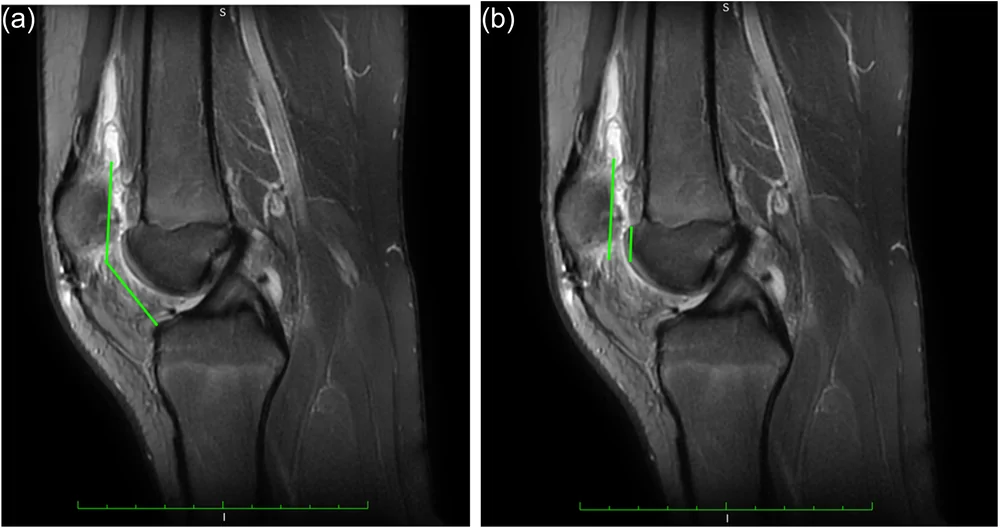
MRI‐sagittal patellar height index (sPHI) (a) and sagittal patellar engagement (SPE) (b) measured on an OPI‐right knee. OPI, objective patellar instability.
